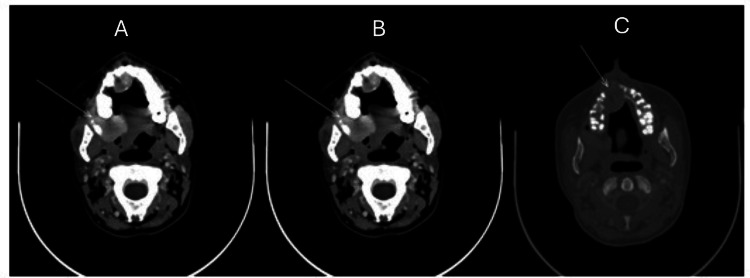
(A-C) Contrast‑enhanced CT of the maxilla showing a 2.6 × 2.2 × 2.1 cm expansile, enhancing destructive lesion (arrows) of the anterior upper jaw with extension through the hard palate into the oral cavity and associated cortical bone destruction. CT: computed tomography
